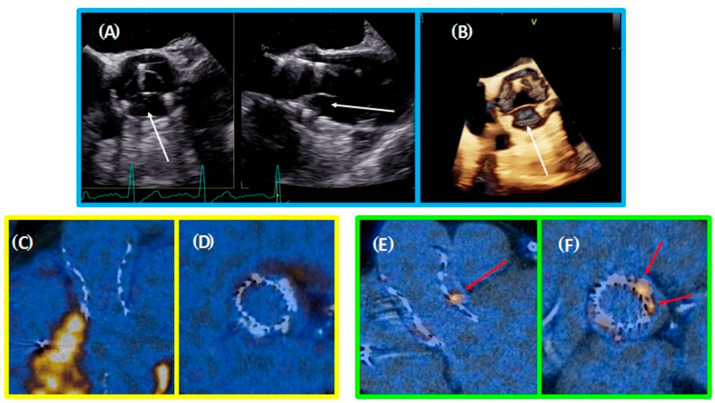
Blue frame: Transesophageal echocardiography (A) multi-D reconstruction: Acurate Neo valve with thrombotic leaflet (white arrows); (B) three dimensional reconstruction: Acurate Neo Valve with marked thrombotic leaflet (white arrow); Yellow frame: [^18^F]F-fluorodeoxyglucose positron emission tomography/computed tomography—no visible uptake at the level of the leaflets; (C) TAVI valve long axis, (D) TAVI valve short axis; Green frame: [^18^F]F-sodium fluoride positron emission tomography/computed tomography- red arrows: visible local uptakes out of the valve (aortic annulus calcifications); (E) TAVI valve long axis; (F) TAVI valve short axis.
